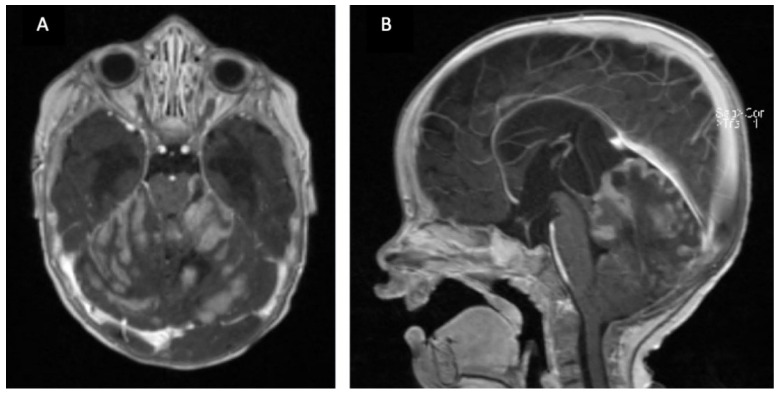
6 month old boy with macrocephaly and developmental delay. Contrasted T1 MRI (A,B) with large cerebellar tumor and associated hydrocephalus. Diagnosis: SHH-medulloblastoma with extensive nodularity (MBEN) WHO grade 4. Gorlin syndrome (SUFU mutation).
